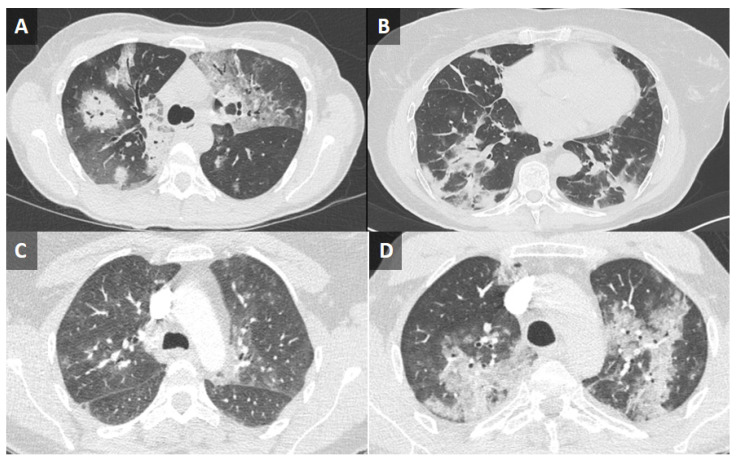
Thoracic computed tomography scan in patients with B-cell depletion and prolonged SARS-CoV-2 infection. (A) Ground-glass interstitial syndrome with a crazy paving, predominant on the left upper lobe, and alveolar condensation in the right lower lobe with pleural effusion (Patient 1). (B) Bilateral ground-glass opacities with sequelae lesions (Patient 2). (C) Subpleural ground-glass opacities with a basal distribution (Patient 3). (D) Multiple bilateral foci of ground-glass opacities, affecting all lobes except the right upper lobe (Patient 4).
